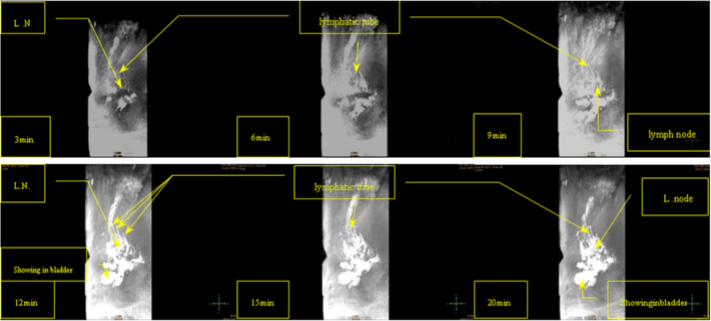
Lymphography (lateral position) Case3:A 42-year-old cervical cancer patient received a slow injection of 20 ml Gadodiamide and 6 ml 2% lidocaine over three minutes. The injection was followed by a 20-minute massage within the MRI system. MR lymphography displays the lymph nodes (L.N.) and lymphatic vessels (L.T.) at various time points. After 12 minutes, the contrast agents appeared mainly in the bladder. There are unmarked elliptically shaped lymph nodes of the iliac blood vessel. The lymphography lateral map was clear. There are numerous unmarked areas with similar shapes to the linear lymph vessels.The lymphatic shape was continuous.
